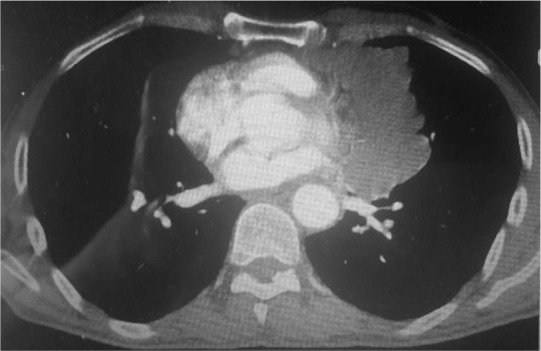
CT scan of patients with thymoma with myasthenia gravis.
